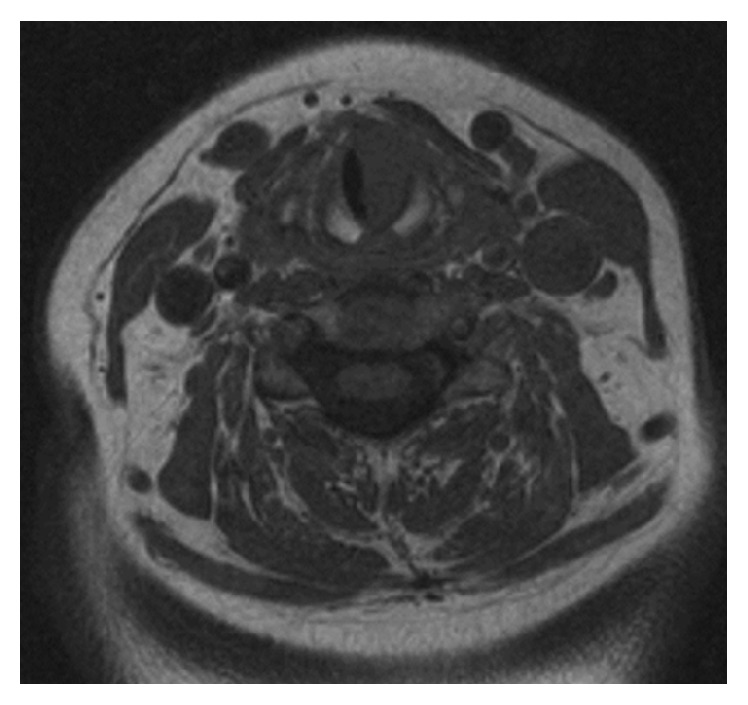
T1-weighted MRI scan showing homogenous soft tissue mass in the left subglottic region.
